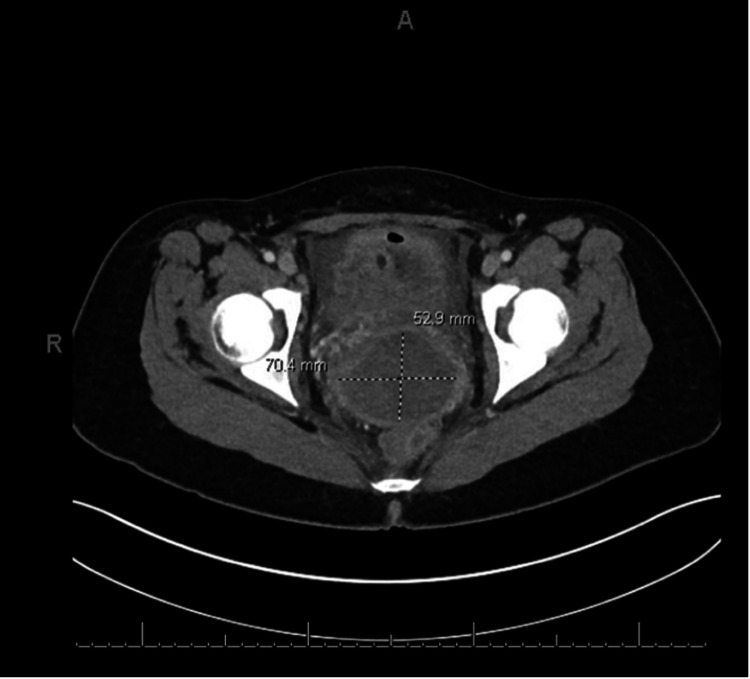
Contrast-enhanced computed tomography findings of cervical and uterine infection Axial image shows measurements of enlarged cervix 52.9 mm x 70.4 mm.
